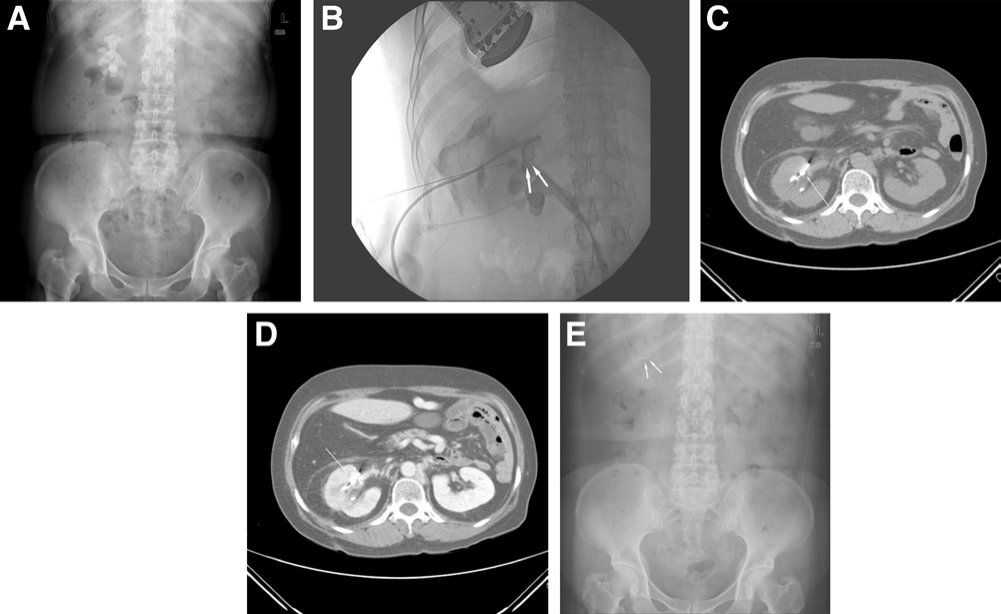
A 54-year-old female patient with a staghorn stone in the right kidney underwent percutaneous nephrostomy (PCN) for percutaneous nephrolithotomy (PNL). (a) The initial kidney, ureter, and bladder (KUB) study image demonstrated a large staghorn stone in the right renal pelvocalyx. (b) A fluoroscopic image obtained during PCN for PNL shows the fractured segment of the guidewire (arrow) at the upper renal calyx of the right kidney. A pigtail catheter was inserted at the upper renal calyx and another yellow sheath was inserted at the lower renal calyx. Leaked contrast media is observed in the right perirenal space. (c) A precontrast computed tomography (CT) image obtained 6 months postoperatively shows the retained fractured guidewire segment (arrow). The fractured fragment appears between the renal parenchyma and renal pelvis. (d) A contrast-enhanced CT image obtained 6 months postoperatively shows increased perirenal fat stranding and decreased enhancement of the right renal parenchyma indicating an inflammatory condition. The fractured fragment (arrow) appears between the renal parenchyma and renal pelvis. (e) The KUB study image obtained 5 years postoperatively shows the retained fractured guidewire segment (arrow).
